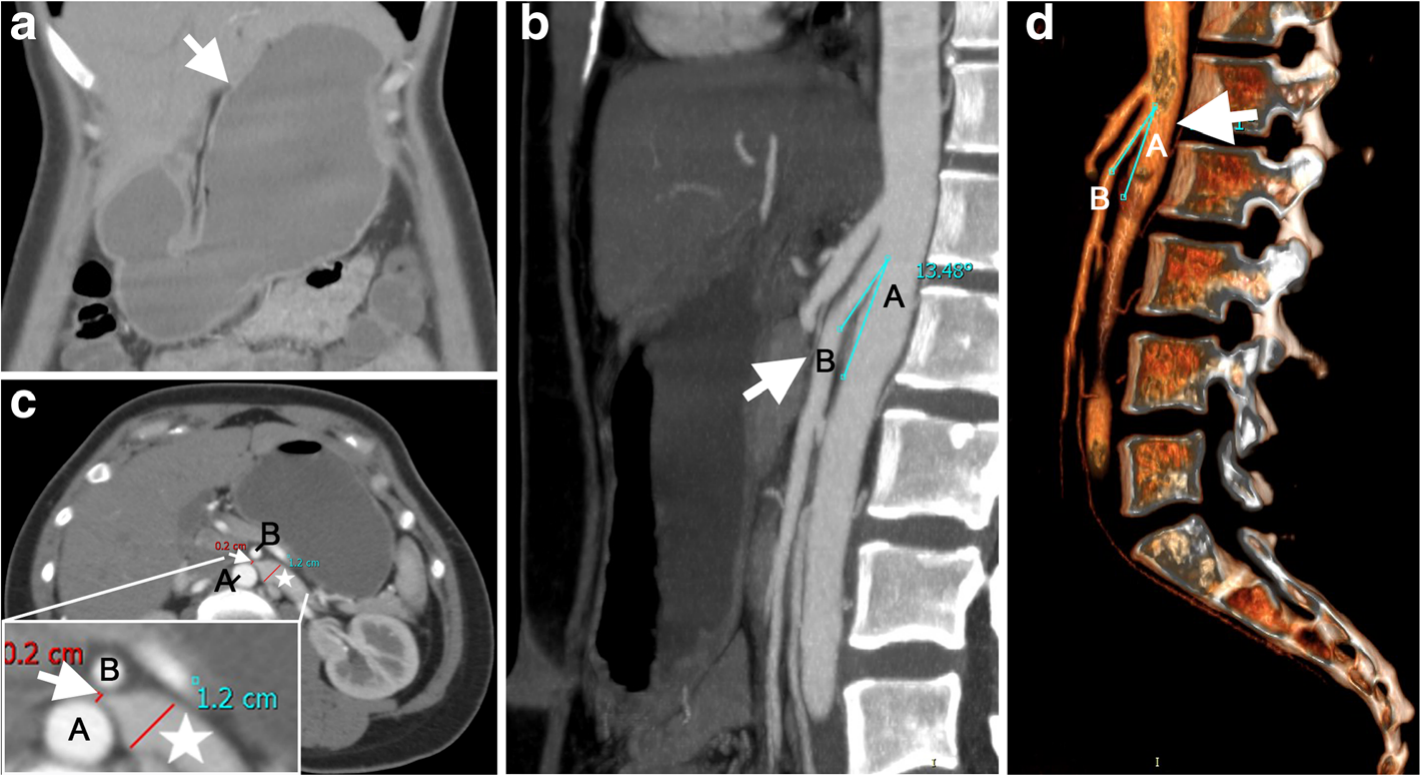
CT imaging showing SMA syndrome and Nutcracker Syndrome. (a) Coronal view of gastroduodenal dilation (arrow). (b) Sagittal view of aortomesenteric region. The angle between the AA “A” and the SMA “B” was less than 35° (measured at 13°) (c) The third portion of the duodenum was compressed by the decreased aortomesenteric angle in the transverse plane. The compressed LRV between the AA “A” and the SMA “B” was showed as arrow (bird beak sign). The dilation LRV was showed as star. The zoomed structures presented in the lower left. (d) Three-dimensional reconstruction of the AA “A” and the SMA “B”
